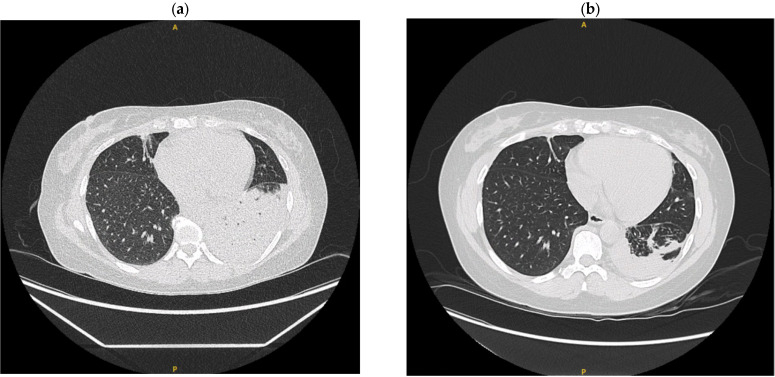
Evolution of chest CT imaging in Case 2: (a) before treatment—December 2023, (b) after treatment—April 2024. Improvement in left lower lobe consolidation with moderate left pleural effusion. A, anterior; P, posterior.
